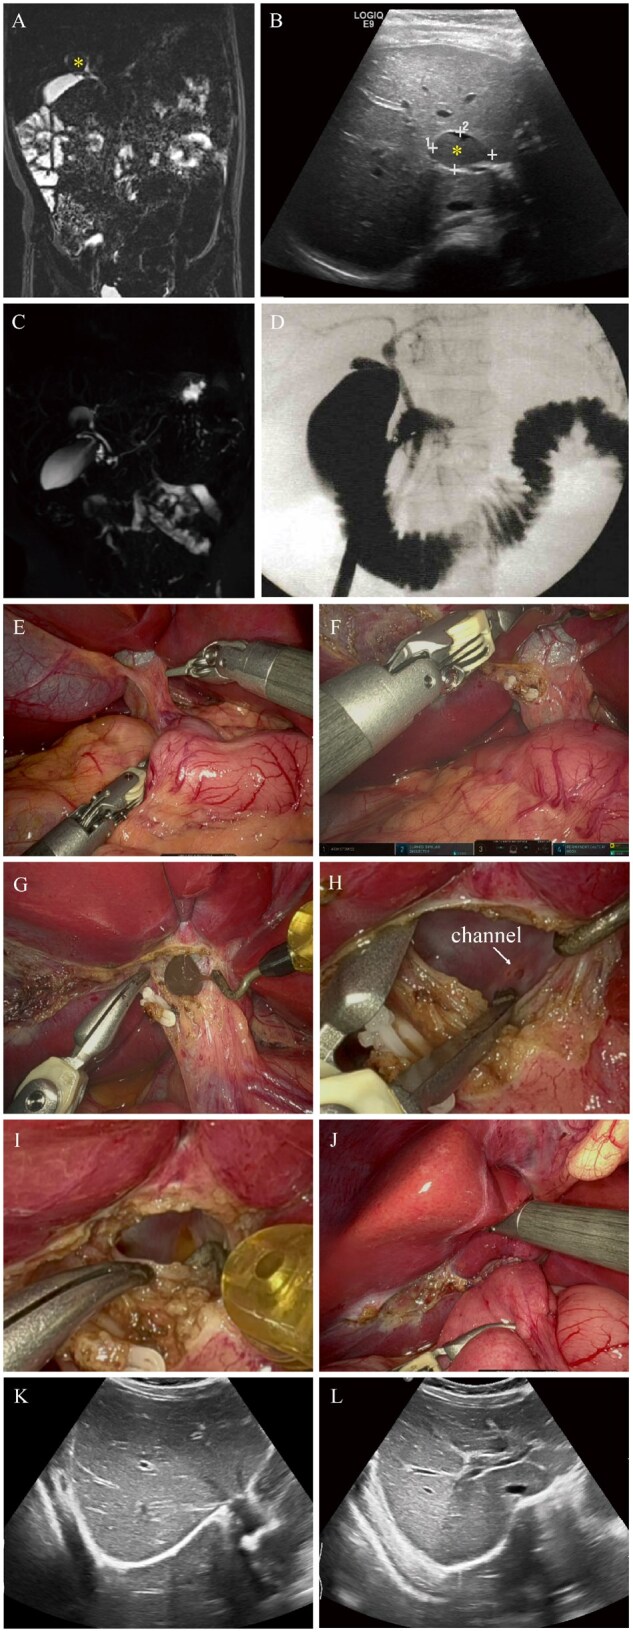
Imaging studies (abdominal ultrasound and MRCP) and intraoperative photographs of case 1. (Α) MRCP before surgery, indicating a round lesion near the hepatic portal vein, measuring approximately 1.9 cm × 1.4 cm and characterized by short T1 and long T2 signals. (Β) Abdominal ultrasound before surgery, indicating a cystic echo near the intrahepatic portal vein, which had increased in size to 2.1 cm × 2.1 cm × 1.3 cm. (C) MRCP before surgery. (D) Cholangiogram of the biliary system in case 1. (E) Comprehensive view of the porta hepatis. (F) Excision of the gallbladder. (G) Discharge of a viscous brownish fluid after opening of the cyst. (H) Channel revealed by puncture and enlargement of the cyst. (I) Bile-like fluid flowing slowly from the channel into the cyst cavity. (J) Anastomosis of the jejunal biliary branch of a right hepatic duct cyst. (K and L) Abdominal ultrasound after surgery, indicating no significant dilation of the intrahepatic or extrahepatic bile ducts.*: cyst.
